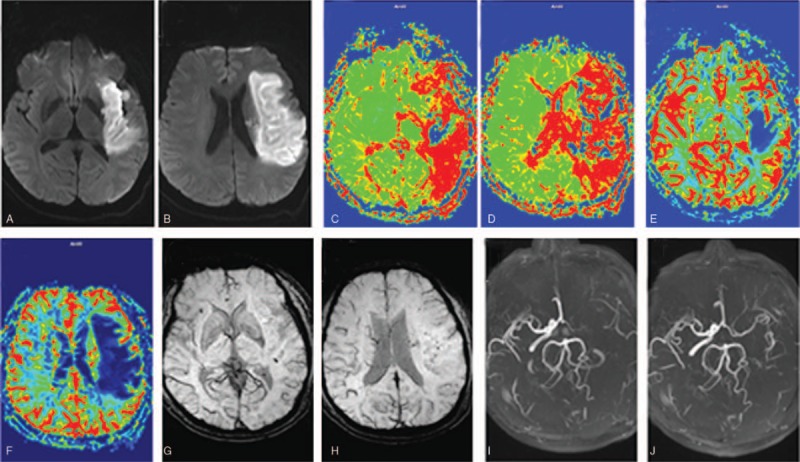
Patient showed cerebral infarction caused by an acute occlusion of the left internal carotid artery. Magnetic resonance angiography (MRA) (I) and diffusion-weighted imaging (DWI) admissions were confirmed (A and B). The National Institutes of Health Stroke scale score at admission was 16 points, and the mean transit time (MTT) at perfusion-weighted imaging (PWI) (C And D) MTT prolonged in the lesion area, MTT - Alberta Stroke Program Early CT score (ASPECTS) was 3 points; in the CWI of PWI (C and E), the cerebral blood volume (CBV) showed a smaller range of increase in CBV than in the healthy side, CBV-ASPECTS was 6 points; in the susceptibility-weighted imaging (SWI) (CWI). (G and H) In the same region, the prominent vein was absent, SWI-ASPECTS was 7 points; after the treatment, the head MRA (J) showed that the left middle cerebral artery was recanalized, and the mRS after 90 days was 4 points. The poor prognosis was reflected in the SWI. On the contrary, the prominent vein grade = 0 suggested that the cortical pia mater was not opened effectively, and although there was revascularization in the later period, the prognosis of the patient was not improved and it was an ineffective revascularization.
